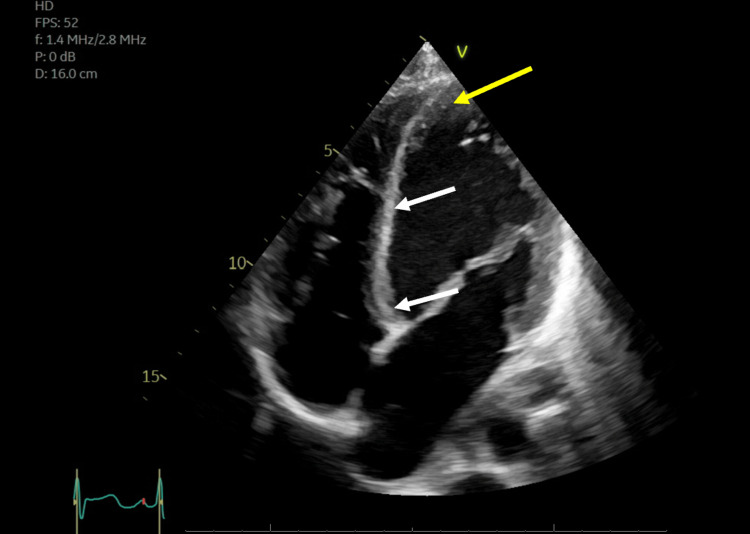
Echocardiogram in ventricular diastole The yellow arrow indicates left ventricular apex during diastole; white arrows indicate left ventricular mid-segment and basilar walls during diastole.
